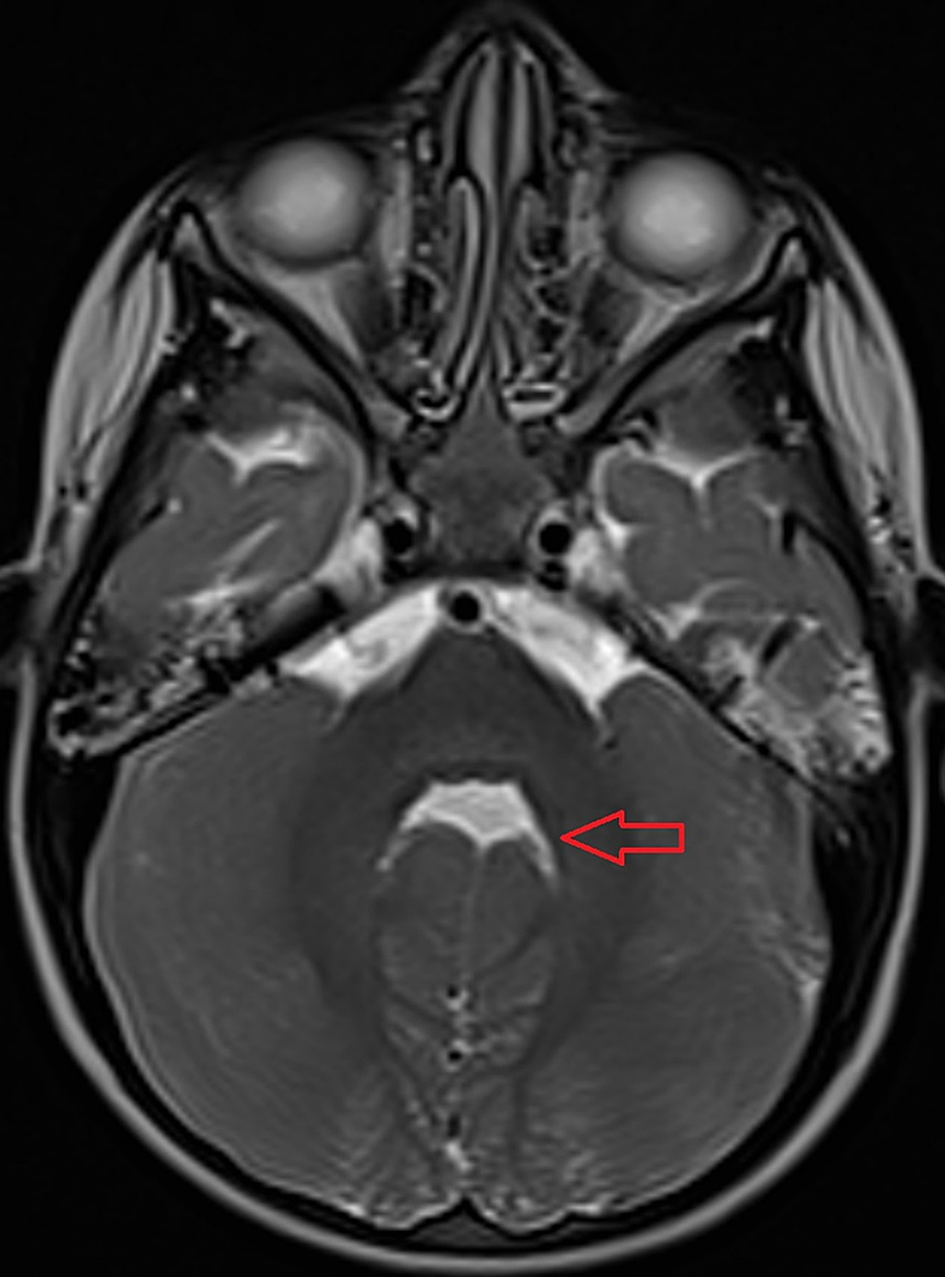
Axial view of brain magnetic resonance image showing absence of the cerebellar vermis results in a bat-wing shaped fourth ventricle (red arrow).
